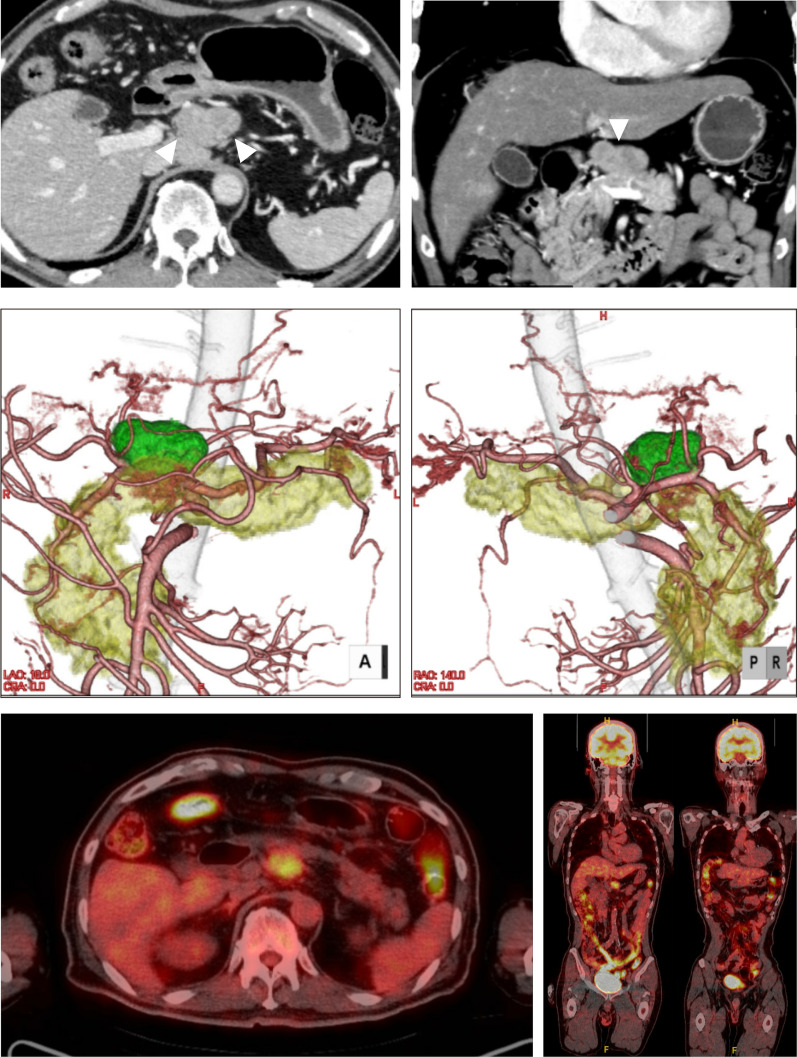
The preoperative contrast-enhanced CT and FDG-PET images. These images showed metastatic tumor in No.8a LN (top), 3D images containing vascular reconstruction (middle), and FDG-PET CT images of No.8a LN (bottom, left) and whole body (bottom, right). Arrowheads indicated the tumor contact with pancreas and close proximity to right gastric artery
